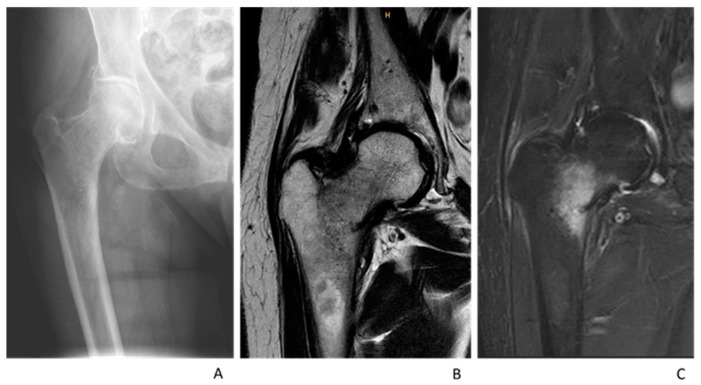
An 87-year-old woman with immobilizing hip pain after low-energy fall. (A): AP Radiograph showing no fracture; (B): T1 Coronar Sequence MRI lateral femoral neck fracture line; (C): STIR Coronar Sequence showing lateral femoral neck oedema.
